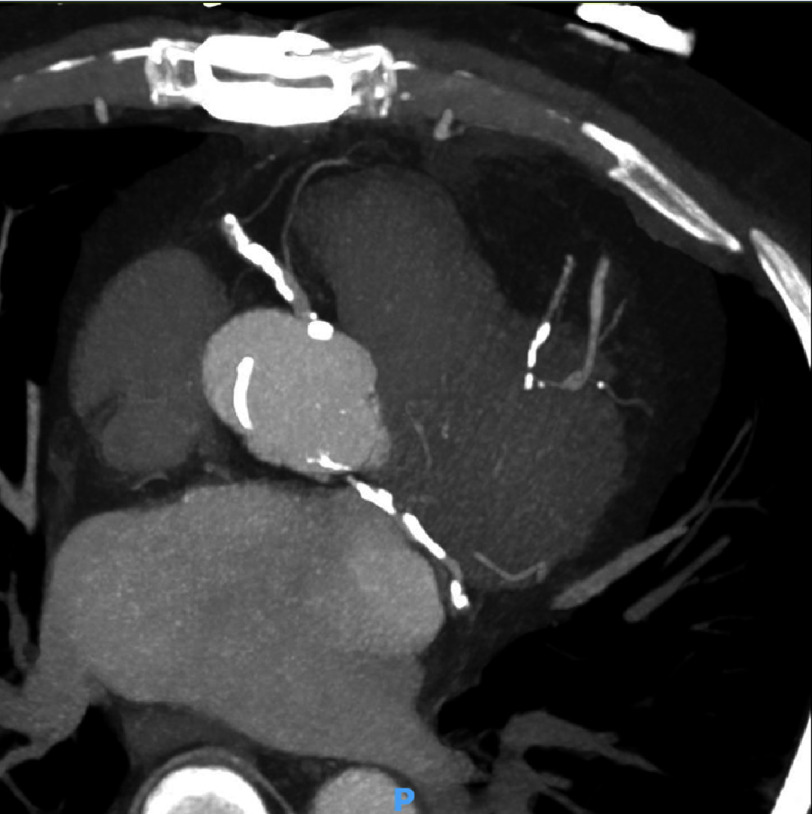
Preoperative axial CTA of heart in patient with prior concomitant CABG and bilateral lung transplant presenting for redo CABG. Calcification of aorta, RCA, and LCA. Both saphenous vein grafts occluded. Abbreviations: CT angiography (CTA), Coronary artery bypass graft (CABG), Right coronary artery (RCA), Left coronary artery (RCA).
